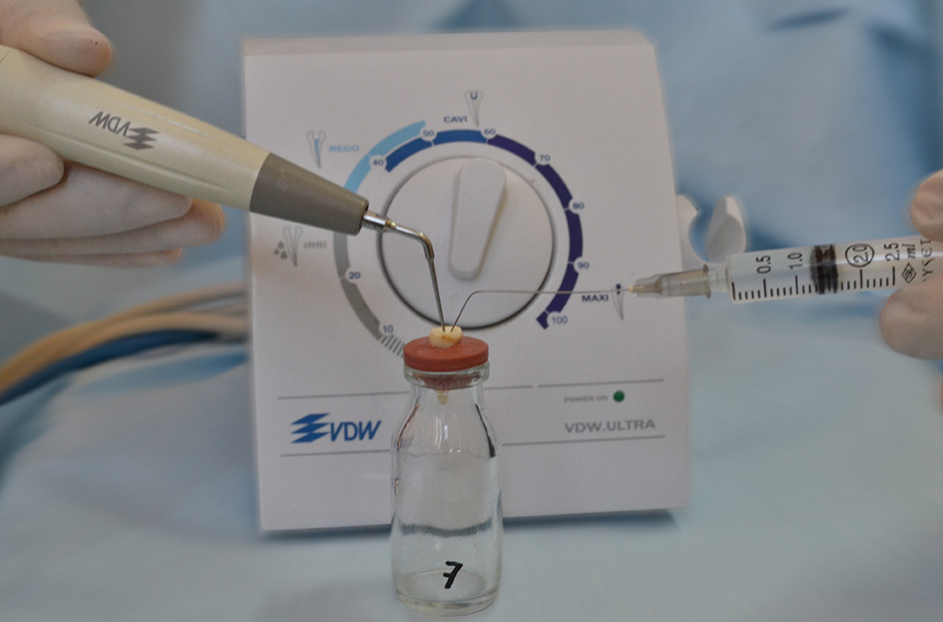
Aqueous Ozone With Ultrasonic Technique
